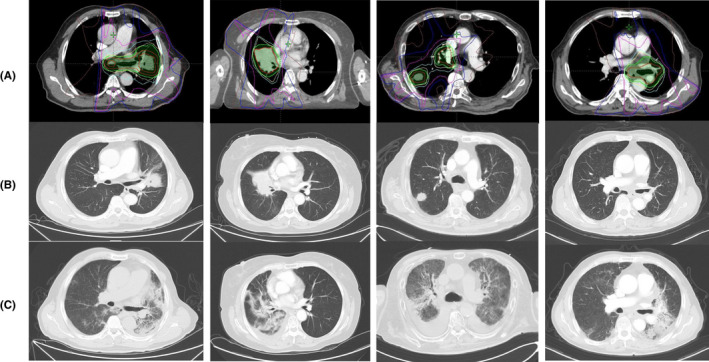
Representative CT images of four patients with symptomatic pneumonitis. (A) Radiation planning imaging with target volume and dose distribution of TRT (B) CT images before TRT; (C) CT images at time of diagnosis of symptomatic pneumonitis
